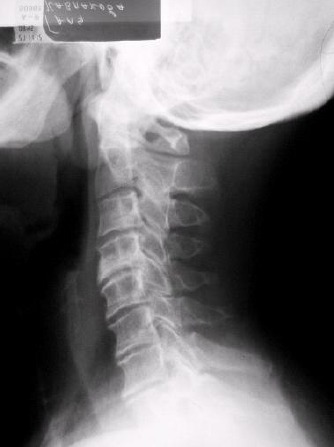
X-ray of the cervical vertebrae in a profile projection*.
